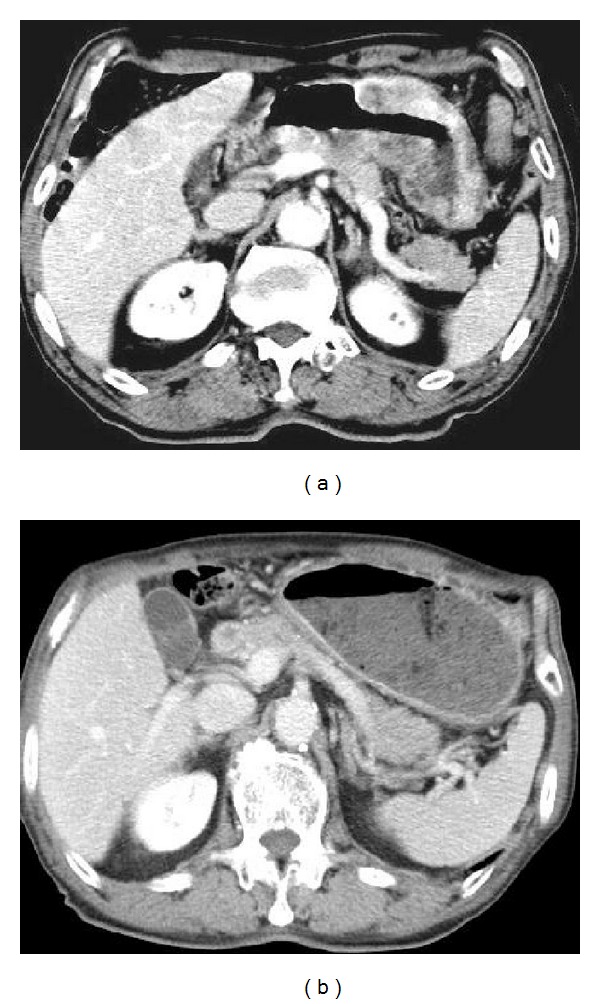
Computed tomography (CT) imaging. (a) The remarkable thickened gastric wall which suggested the invasion of the pancreas was depicted before chemotherapy. (b) Normalized gastric wall and separated gastric wall and pancreas was seen after chemotherapy.
